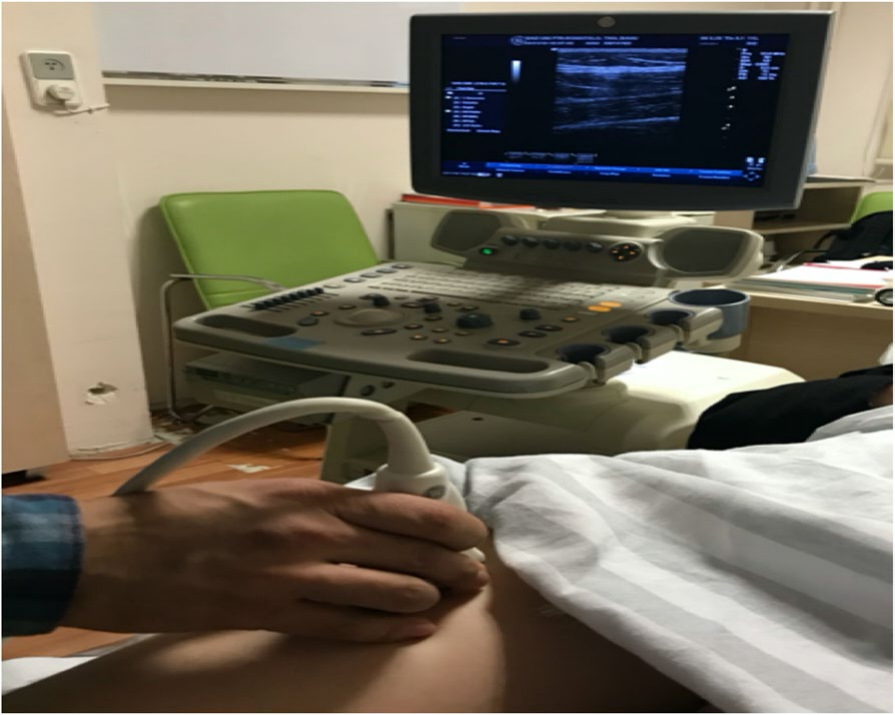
Rectus femoris—vastus intermedius ultrasonographic evaluation
